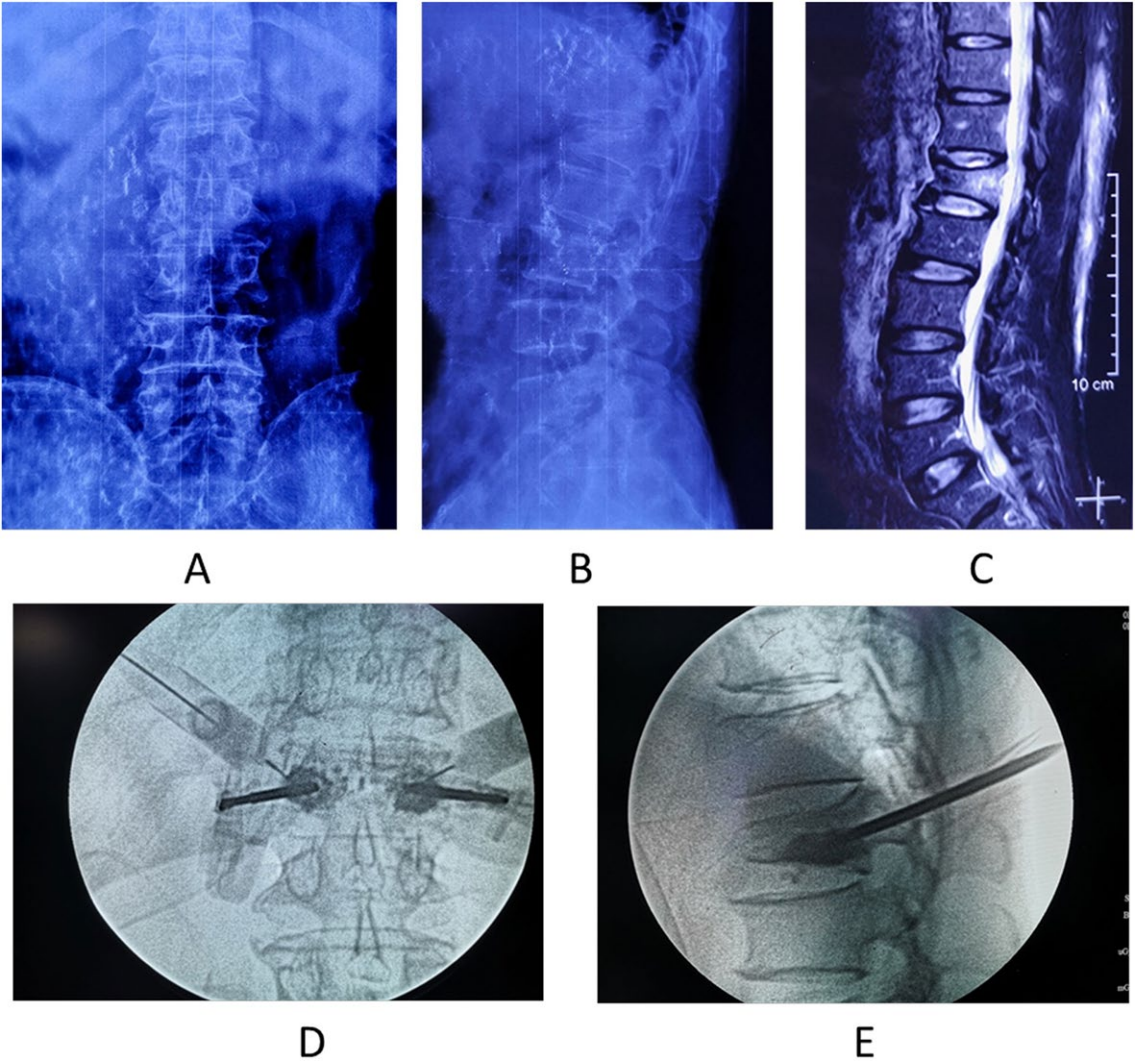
A B Anteroposterior and Lateral view shows the compressed L1 vertebra. C Short tau inversion recovery sequences magnetic resonance image. D E Anteroposterior and Lateral view shows the needle was inserted the juncture of the superior articular process and transverse process
